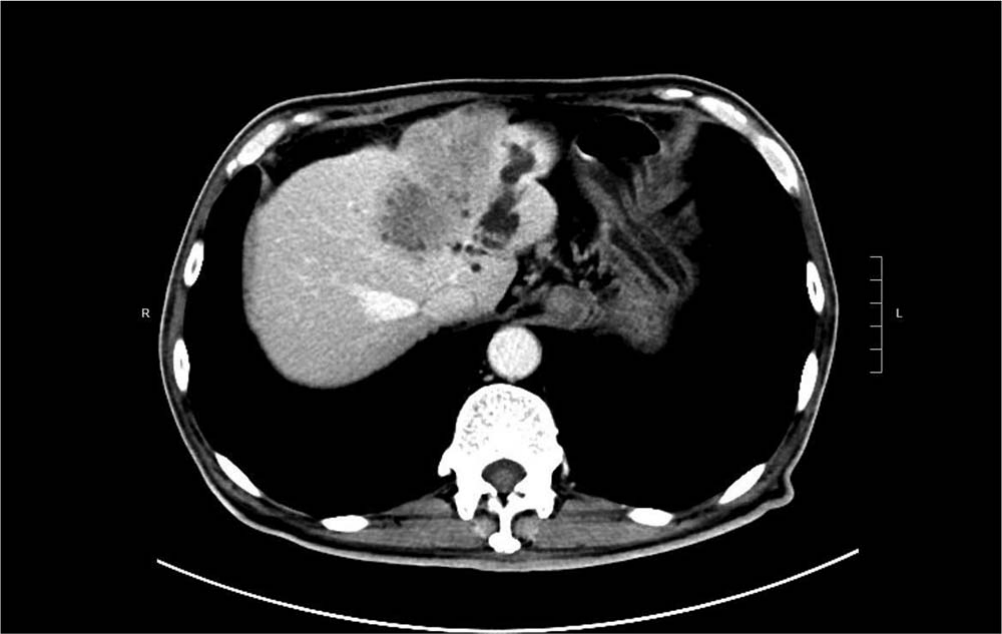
CT showing showing an exophytic hypoenhancing mass at left liver with dilated left intrahepatic duct.
